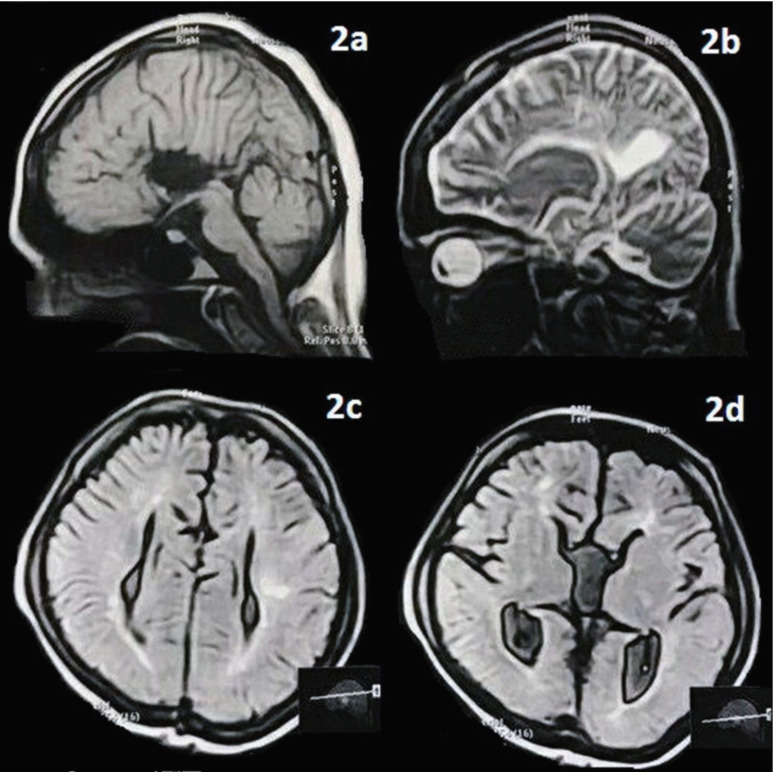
Sagittal T1-weighted (2a) and T2-weighted (2b) MRI of the brain revealed complete absence of corpus callosum and cingulate gyrus and radial arrangement of sulci. Axial Fluid-attenuated inversion recovery (FLAIR) MRI of the brain showed parallel lateral ventricles (2c), “race-car” appearance of lateral ventricles, and colpocephaly (2d). All of these findings suggest Agenesis of the corpus callosum (ACC).
